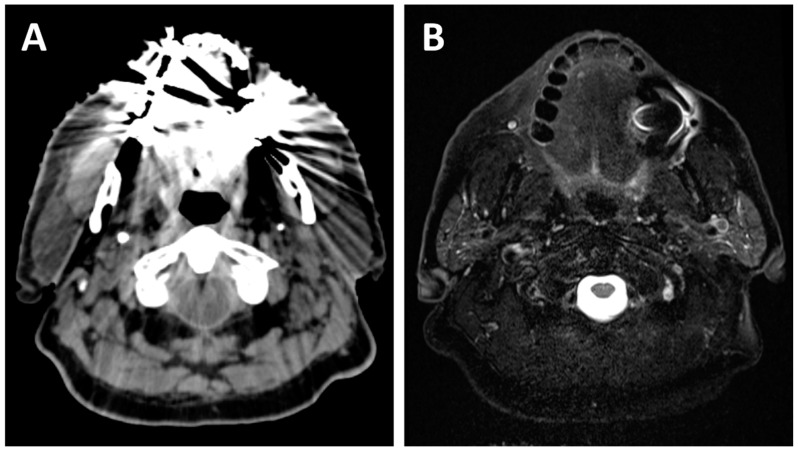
A 70-year-old patient with dental amalgam planned for concurrent chemoradiotherapy for a cT3N2b squamous cell carcinoma of the right base of tongue. The planning CT (A) shows poor image quality with important artifacts which could easily obscure oral or oropharyngeal pathology. The planning T2-weighted MRI (B) also shows artifacts due to dental amalgam, but the image quality remains sufficient for adequate radiological evaluation of most of the oral cavity and oropharynx.
